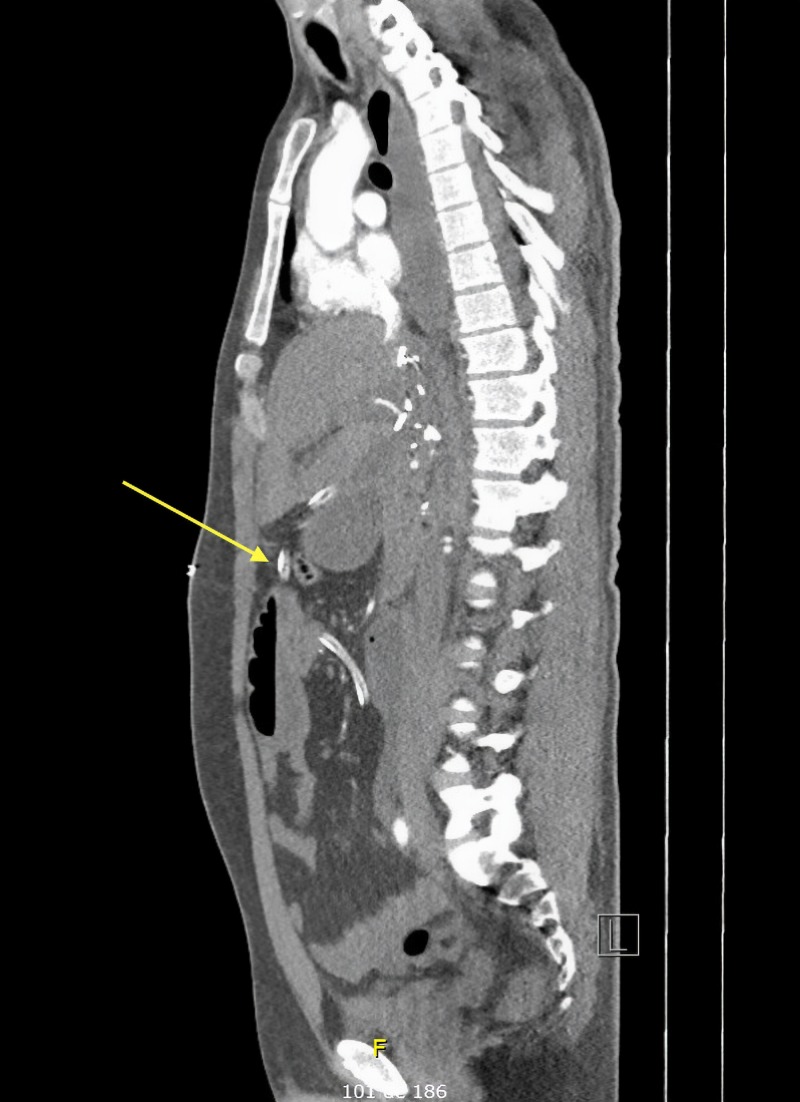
Sagittal view of the CT scan showing, once again, the drain’s course throughout the abdomen.
